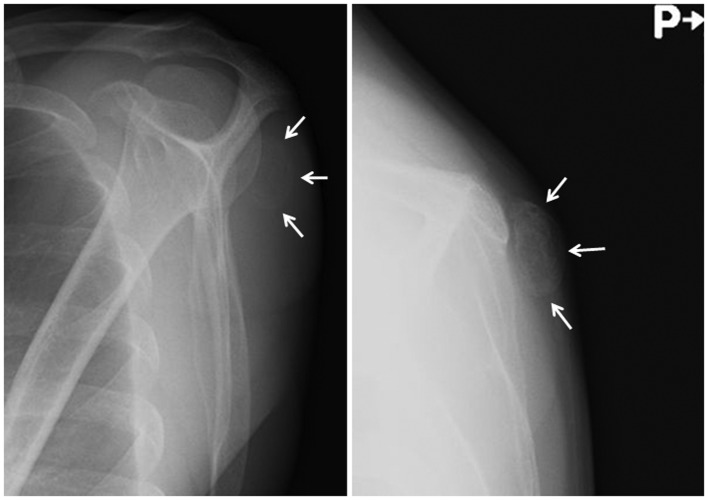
Plain radiographs revealing a faintly ossified soft-tissue mass (arrows), without evidence of bone erosion.
